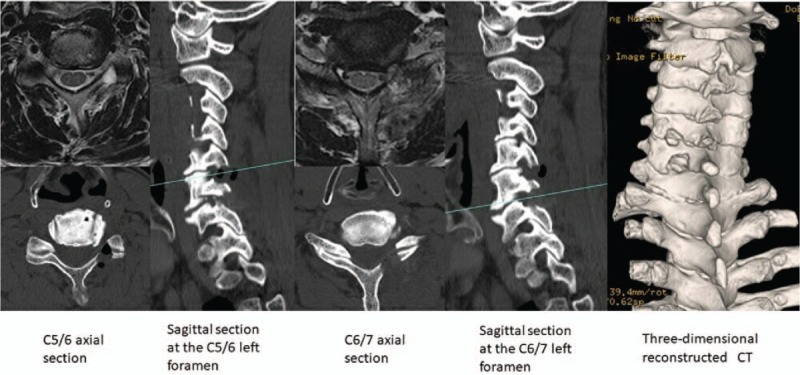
Computed tomography (CT) and magnetic resonance imaging scans (T2 axial slices) after surgery showing sufficient decompression.
